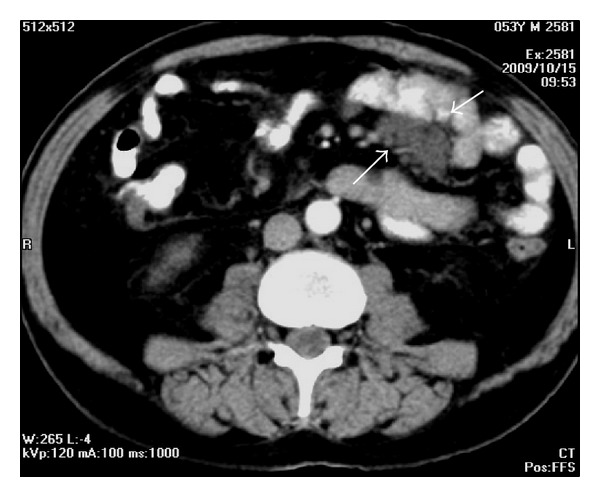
A 53-year-old man with lymphoma. Axial contrast-enhanced CT demonstrates mesenteric lymph nodes.
